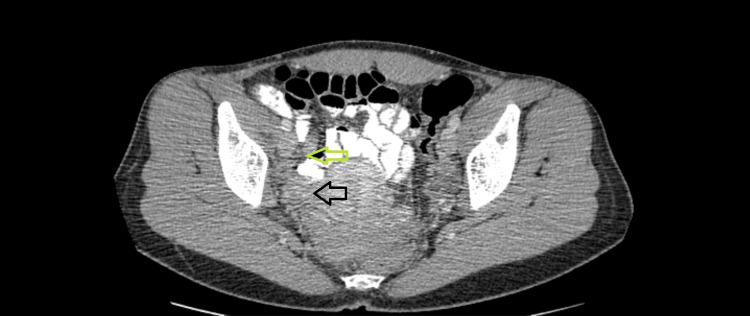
Computed tomography imaging of the abdomen, with intravenous and oral contrast The appendix (green arrow) with signs of inflammation, located next to the right ovary (black arrow)
